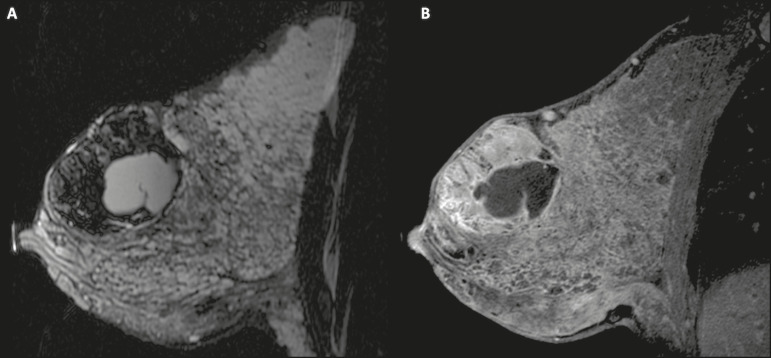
A 34-year-old lactating patient complained of a rapidly growing mass in the left breast. T2 (A) and post-contrast T1 (B) weighted MRI images showed a complex solid and cystic mass in the upper quadrants of the left breast, in the same topography of a previous biopsy compatible with fibroadenoma. A new percutaneous biopsy confirmed the diagnosis of fibroadenoma with lactational changes.
